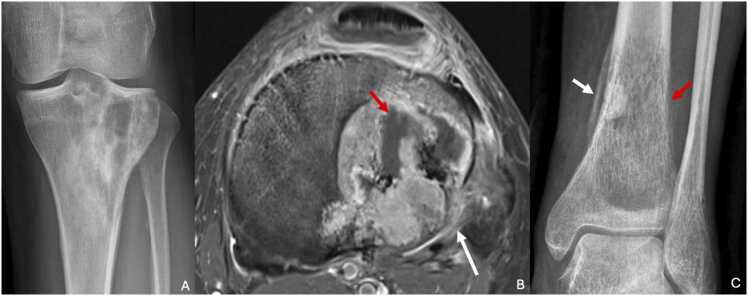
A,B. Osteosarcoma in a 42-year-old woman with left gonalgia for six months. C. Ewing sarcoma of the left tibia in a 45-year-old woman incidentally discovered after a trauma (different patient). A. Face radiography showing a heterogenous lesion of the proximal third of left tibia with blurred boundaries. B. T1-weighted fat-suppressed contrast-enhanced MR image in the axial plane showing an intense enhancement of this tumor with necrotic central areas (red arrow). Notice adjacent osteomedullar edema, periosteal reaction and extraosseous extension (white arrow). C. Face radiography showing a large osteolytic lesion with lytic cortex (red arrow) and plurilamellar periosteal reaction (white arrow).
